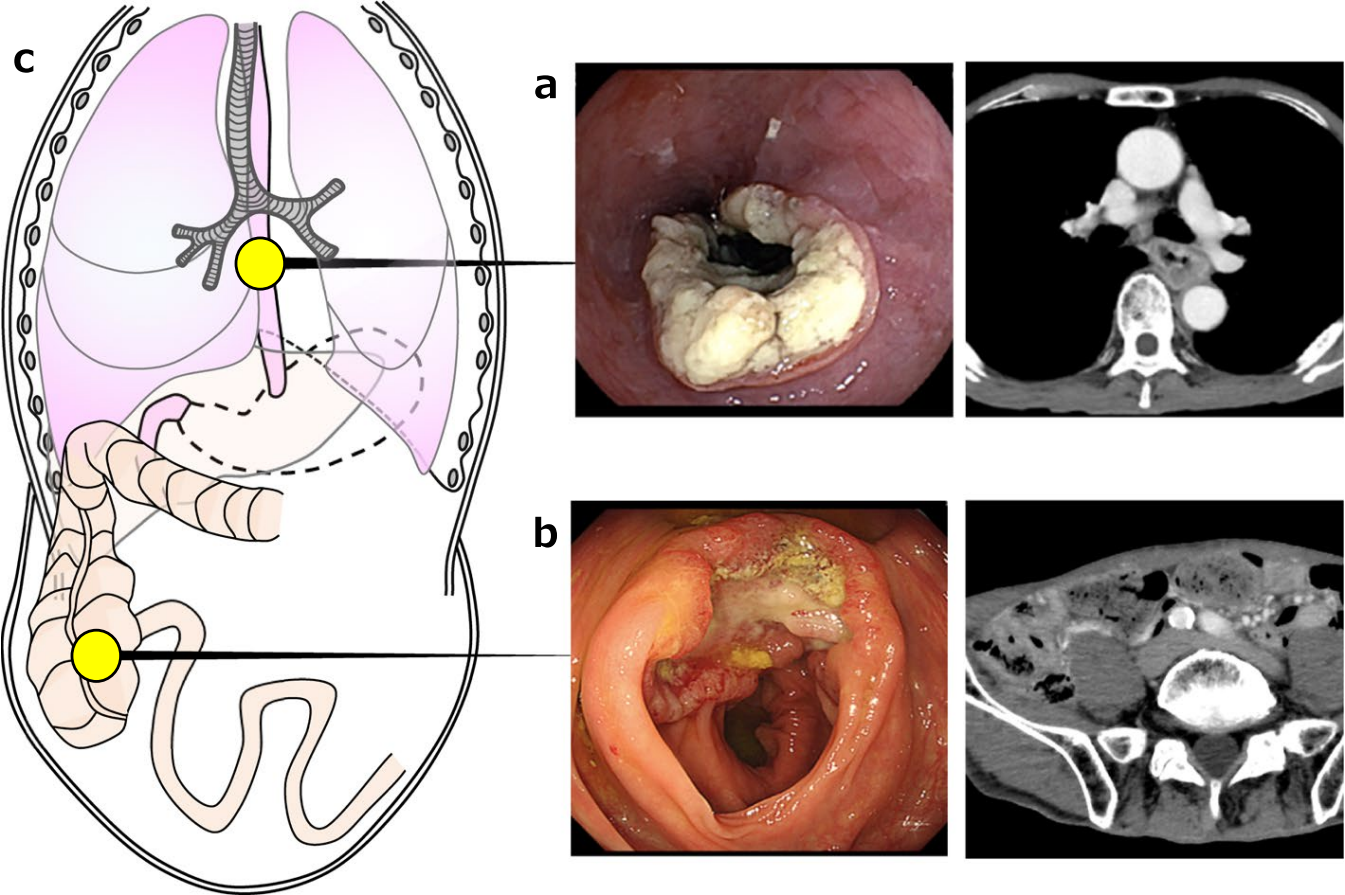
Preoperative endoscopy and contrast-enhanced computed tomography findings. a Esophageal squamous cell carcinoma T3N1M0 in the median thoracic esophagus. b Cecal cancer T3N0M0. c Prior to the start of treatment, the method of reconstruction after total gastrectomy was unknown
